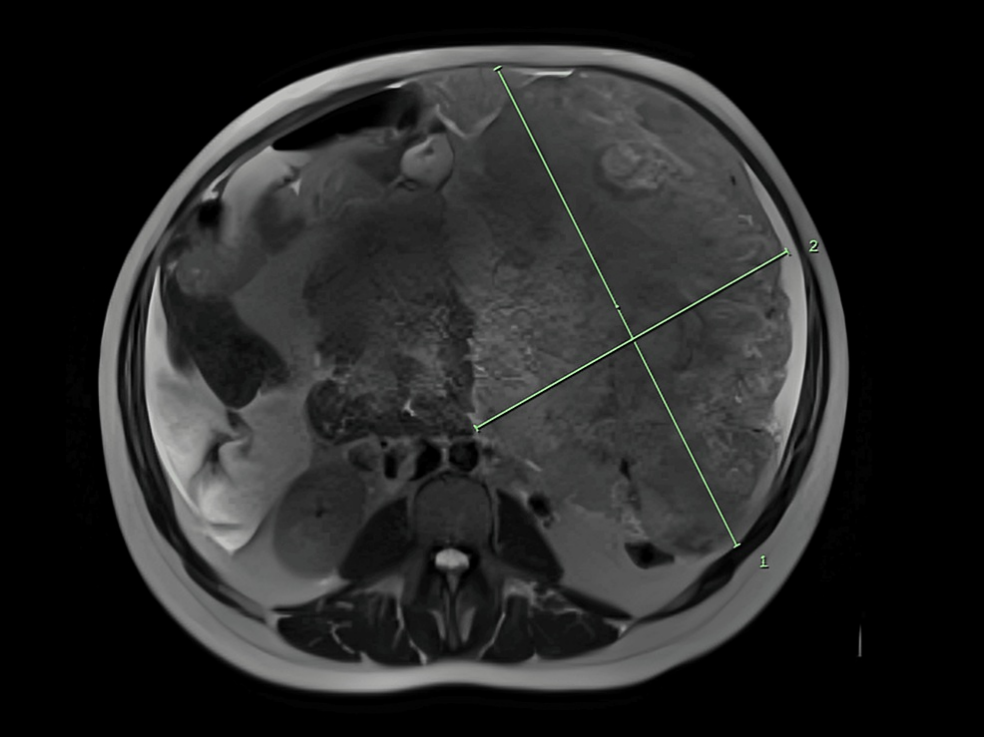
MRI T2 axial plane demonstrating a complex left upper abdominal mass with a maximum diameter of 26 cm.
